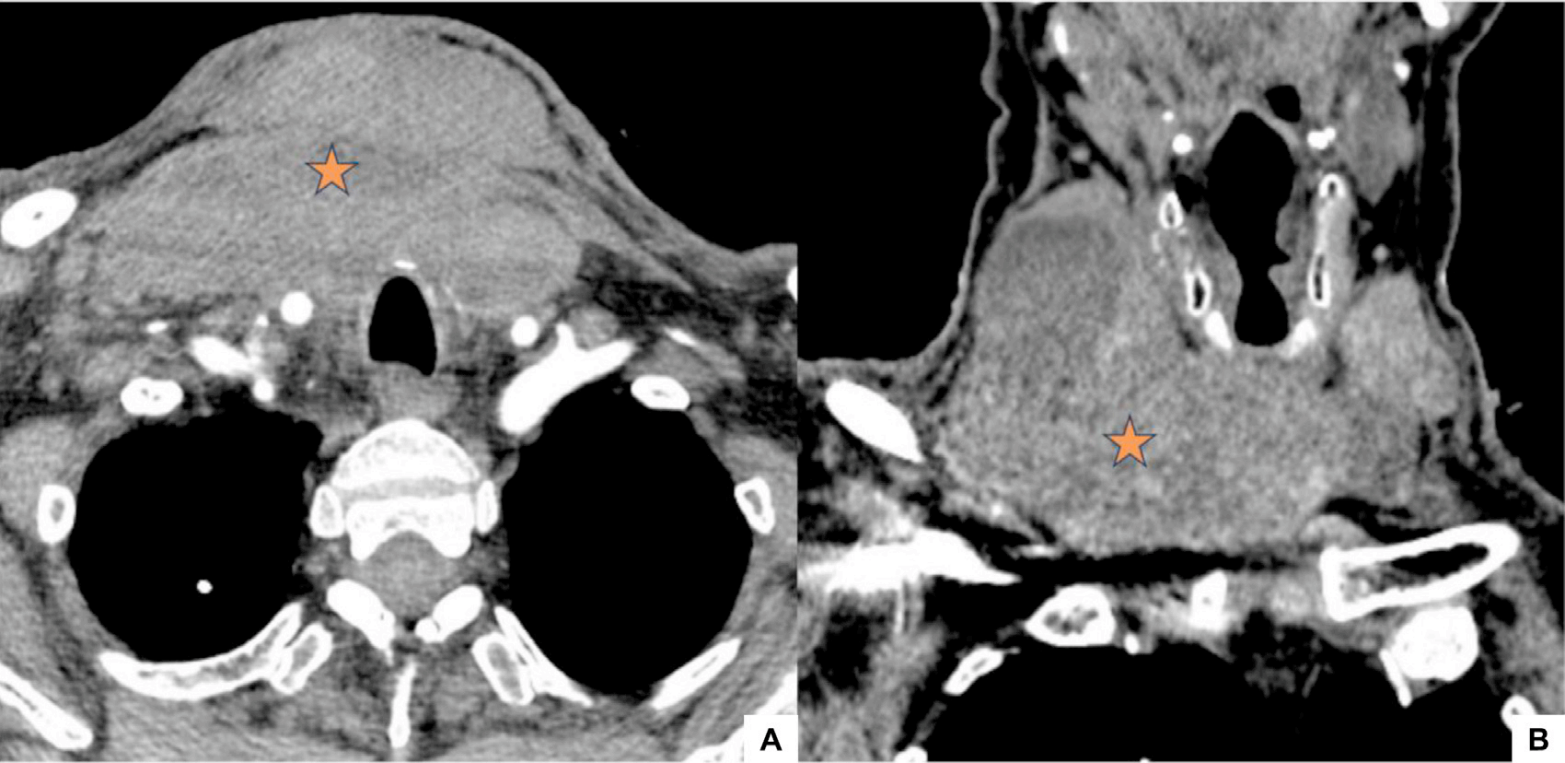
Axial (A) and Coronal (B) cervical CTA images after revision surgery: Significant increase in hematoma volume (asterisk), causing slight tracheal deviation, with no signs of active bleeding in the hemorrhage site.
